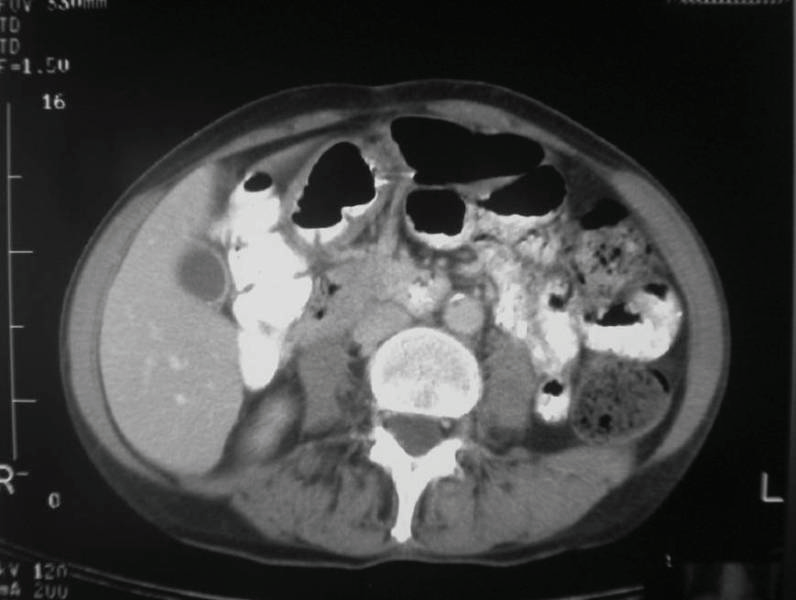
Follow up abdominal CT scan of the same patient after one month. There aren’t any pathologic findings.
